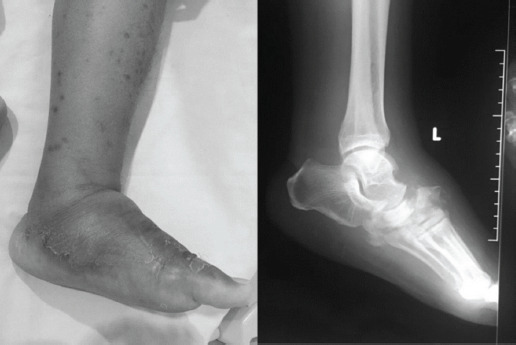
Clinical picture and radiograph of a diabetic Charcot arthropathy patient with limited ankle dorsiflexion due to Achilles tendon contracture.
